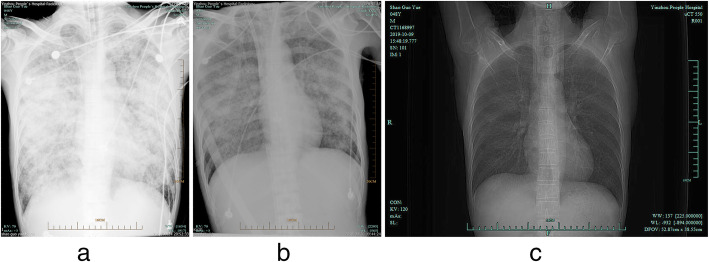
a: Chest X-ray before ECMO treatment. Diffuse exudation was observed in both lungs, presenting as a large white lung. b: Chest X-ray on day 6 of ECMO treatment. The diffuse exudation in both lungs was significantly absorbed compared with the image captured at admission. c: Chest X-ray on the 9th day after the removal of ECMO. The pneumonia was completely absorbed without residual disease
